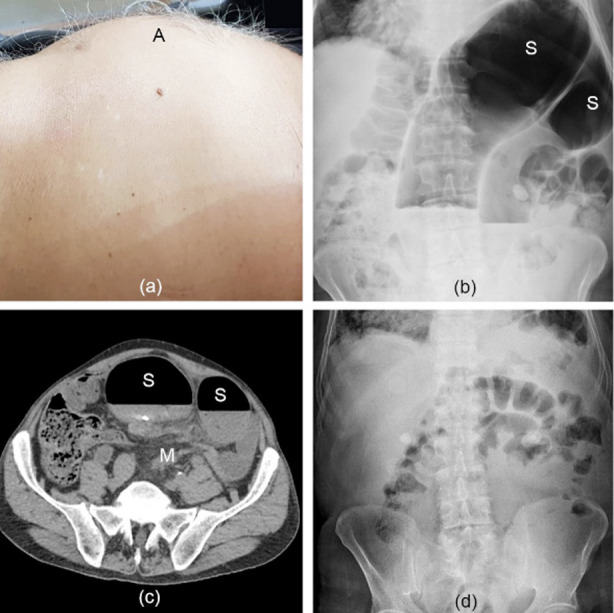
(a) Clinical appearance (A: asymmetrically distended abdomen), (b) Plain abdominal X-ray image (S: twisted sigmoid colon segments forming coffee bean sign), (c) Axial abdominal CT image (S: dilated sigmoid colon, M: mesenteric whirl sign), (d) Control plain abdominal X-ray image.
